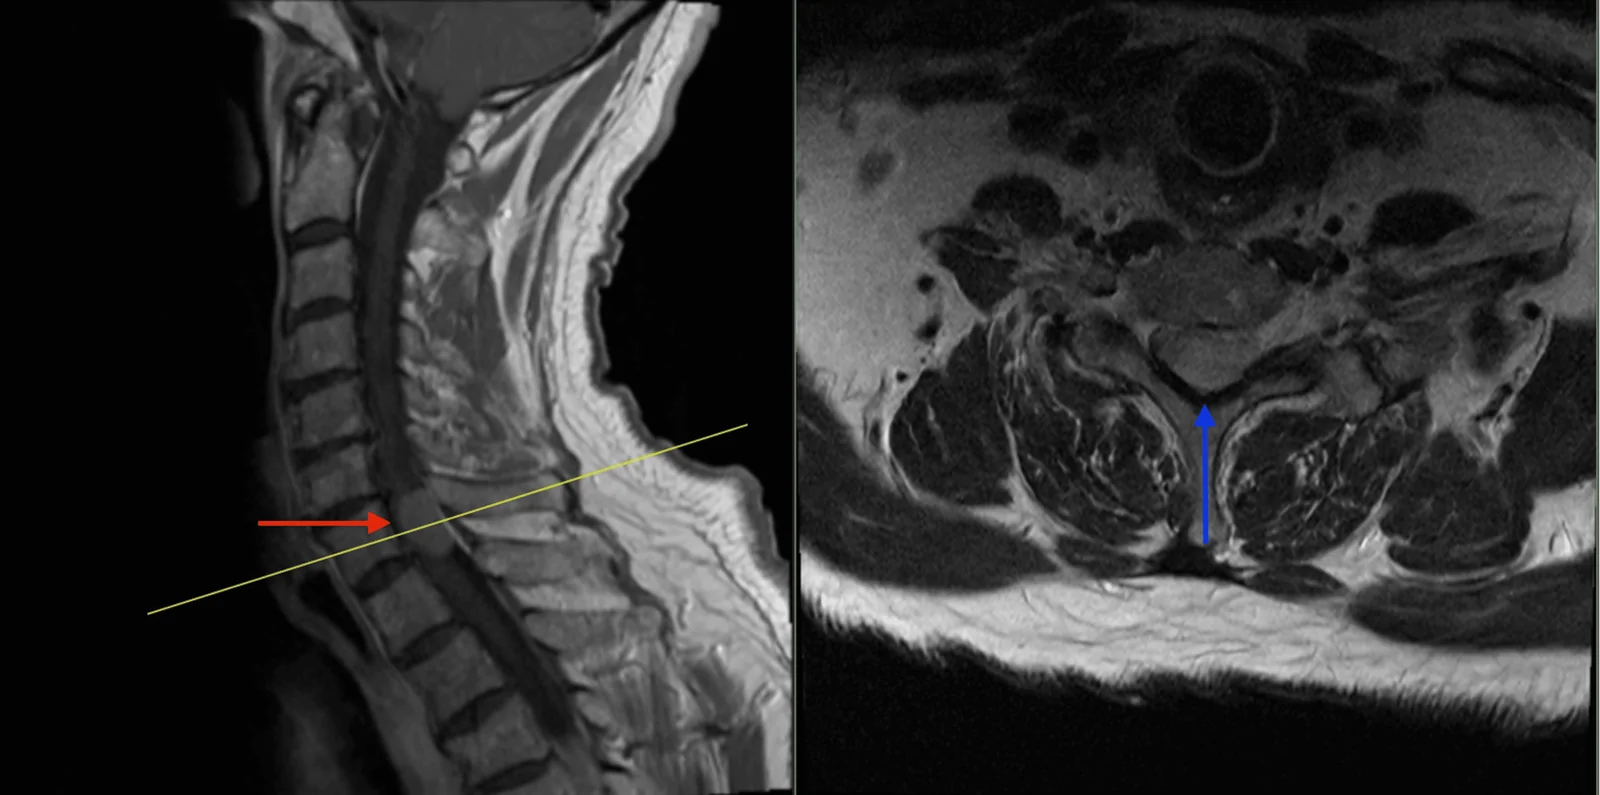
T1 with contrast spinal MRI with meningioma in axial view Sagittal (left) and axial (right) T1-weighted cervical spine MRI with contrast demonstrating an intradural extramedullary mass on the axial view, consistent with a meningioma, causing displacement of the cervical spinal cord. The sagittal slice position is indicated by the yellow line.
